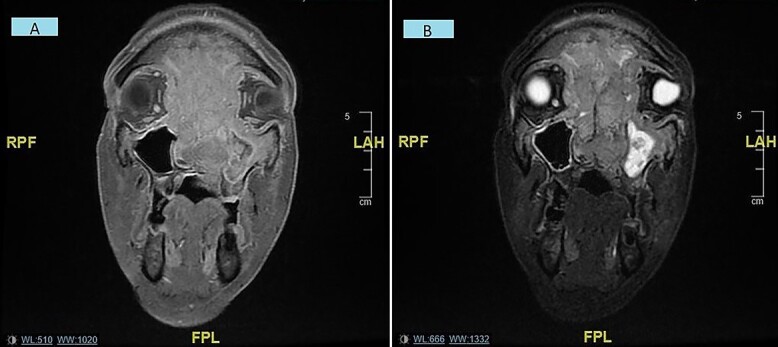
(A and B) Coronal MRI sinuses/head images displaying sinonasal mass invading anterior skull base, orbits and intracranially/intradurally (2A—T1 weighted, 2B—STIR).
